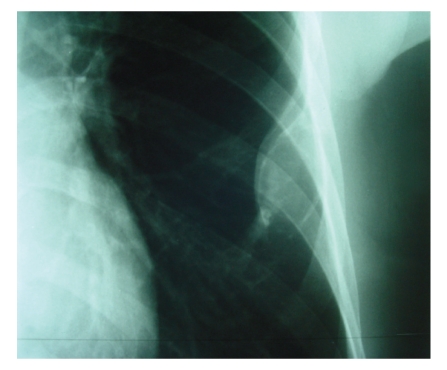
Chest radiography : Ovoïd opacity of the lateral arch of the 6th left rib.
